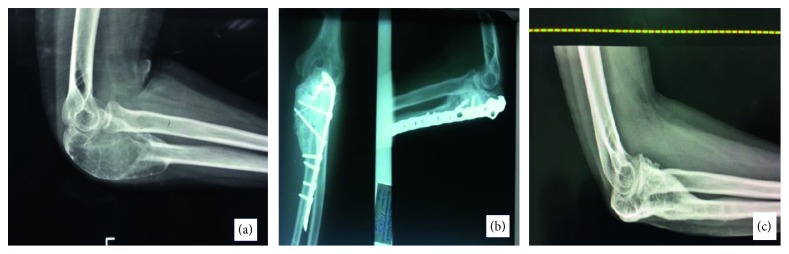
(a) Lateral elbow X-ray showing expansile cystic lesion involving the whole olecranon. (b) AP and lateral elbow X-rays after surgery with olecranon anatomical locking plate. (c) Lateral X-ray 4 years after removal of metal showing complete healing of the lesion.
